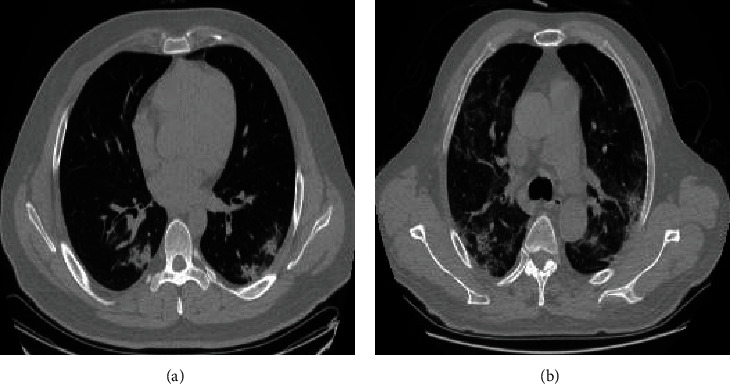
Examples of incorrectly and correctly classified CT images. (a) represents incorrectly classified image. (b) represents correctly classified image.
